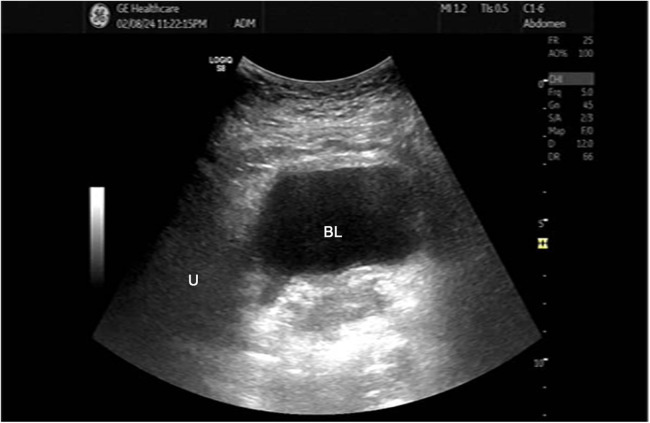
Postoperative bladder re-examination. The bladder was evaluated by color ultrasound. U, uterus; BL, bladder.
